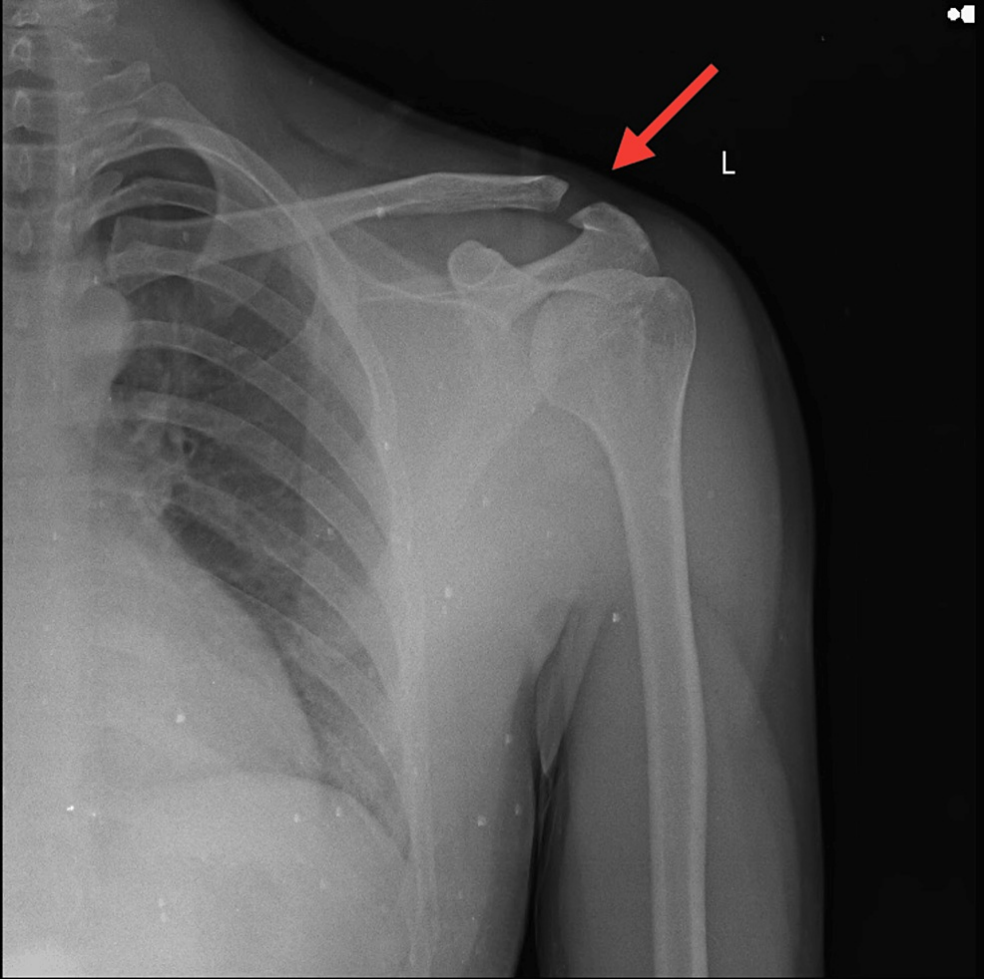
Radiograph of left shoulder showing grade 1 AC joint sprain AC, acromioclavicular
